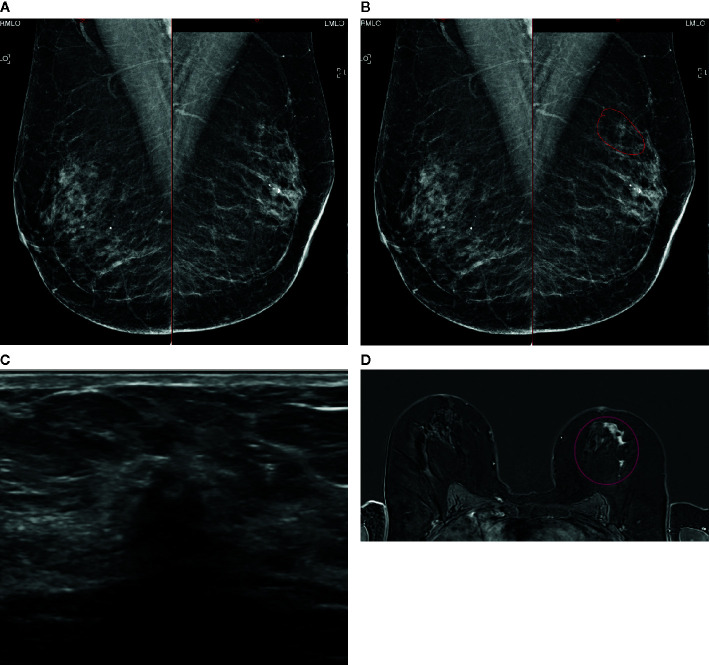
A case of extensive ILC in a 50-year-old female attending for 1^st^ breast screening. (A) Mammogram: Right and left MLO views. (B) Mammogram is reported as M3. There is a 5-mm area of concern on the left which is circled. The calcifications are considered benign. The patient does not feel a palpable mass. (C) An US of the left breast is performed and shows a small 8mm focus close to the nipple. (D) MRI breast follows and this shows an extensive area measuring approximately 50 mm × 50 mm (circled). Biopsies were performed and showed Gr2 ILC ER8 PgR8 HER negative. Patient had mastectomy and sentinel node biopsy (SNB) that showed 52-mm Gr2 ILC ER8 PgR8 HER2 negative SNB 0/3. (A) Mammogram: Right and left MLO views (B) Mammogram M3: possibly a 5-mm area of concern on the left (circled). Calcifications look benign. (C) Ultrasound: One small focus closer to nipple maybe 8mm. (D) MRI: Extensive central disease over 50 mm × 50 mm (circled).
